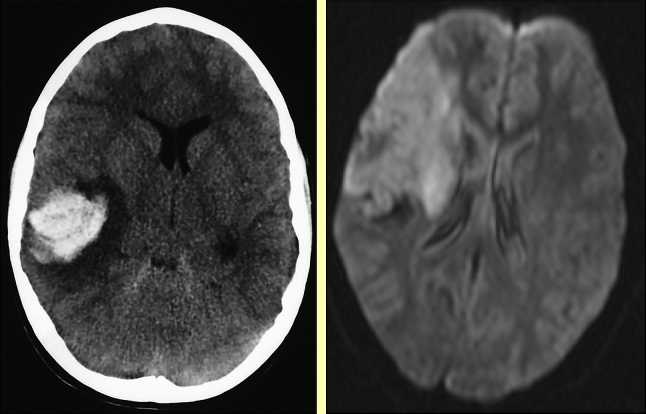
Emergency imaging in unilateral stroke. Left: CT showing acute intracerebral haemorrhage with surrounding focal oedema in a child presenting in coma after a focal seizure, Right: MRI showing infarction in the territory of the occluded middle cerebral artery in a child with a dense hemiparesis; the CT scan had been reported as normal although there was subtle abnormality.
